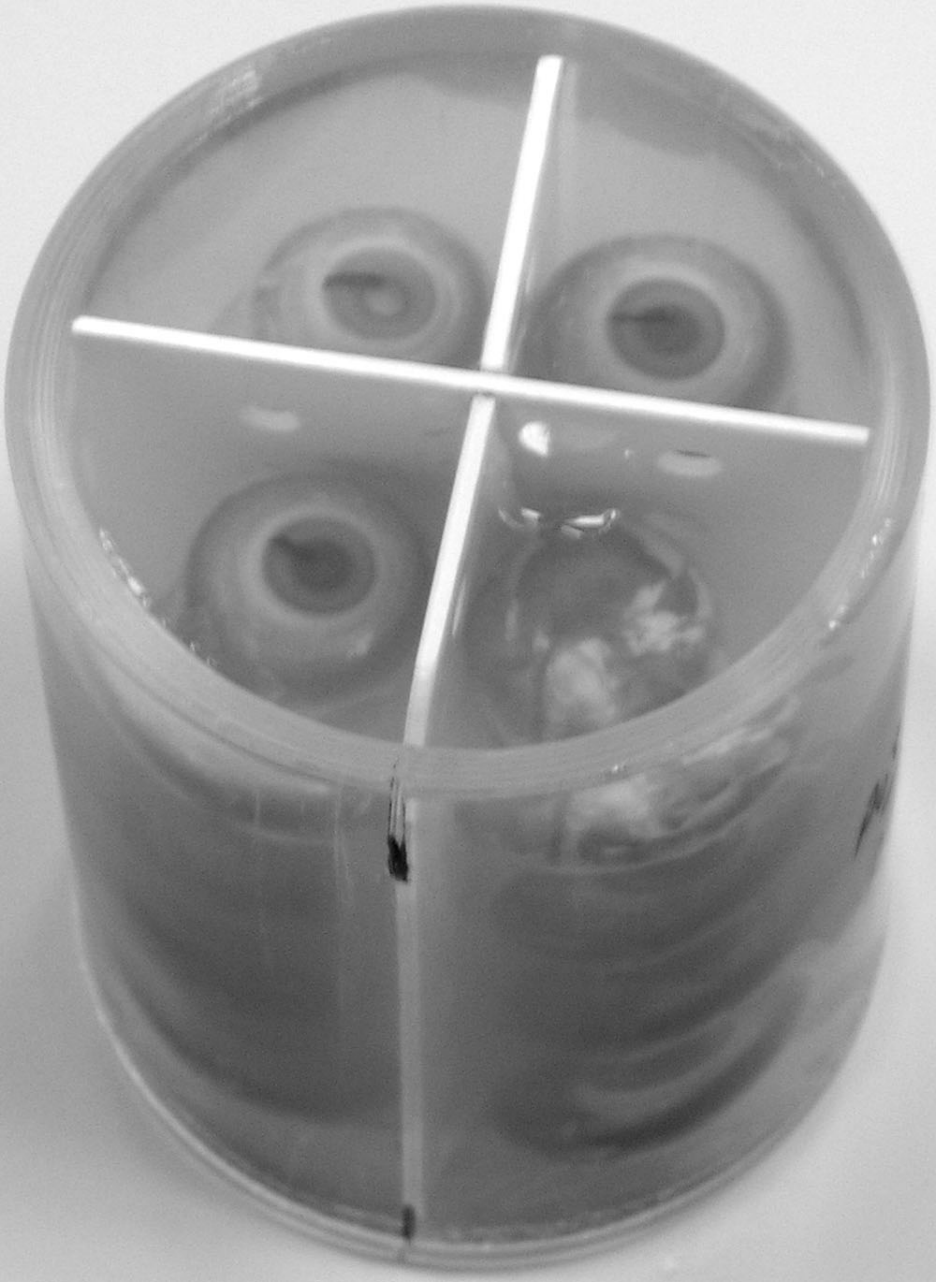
Arrangement of eyes for magnetic resonance imaging scanning. The figure shows 16 paraformaldehyde-fixed chicken eyes embedded in low-melting-point agarose in a 2×2×4 array in readiness for an overnight magnetic resonance imaging (MRI) scan. Note that the eye in the lower-right quadrant of the uppermost layer was positioned in an inverted orientation to permit unambiguous identification of each eye in the resultant MRI image. In the remaining three eyes of the uppermost layer, it is possible to see the ink mark on the nasal cornea, which was used to indicate the original orientation of the eye in the head.
